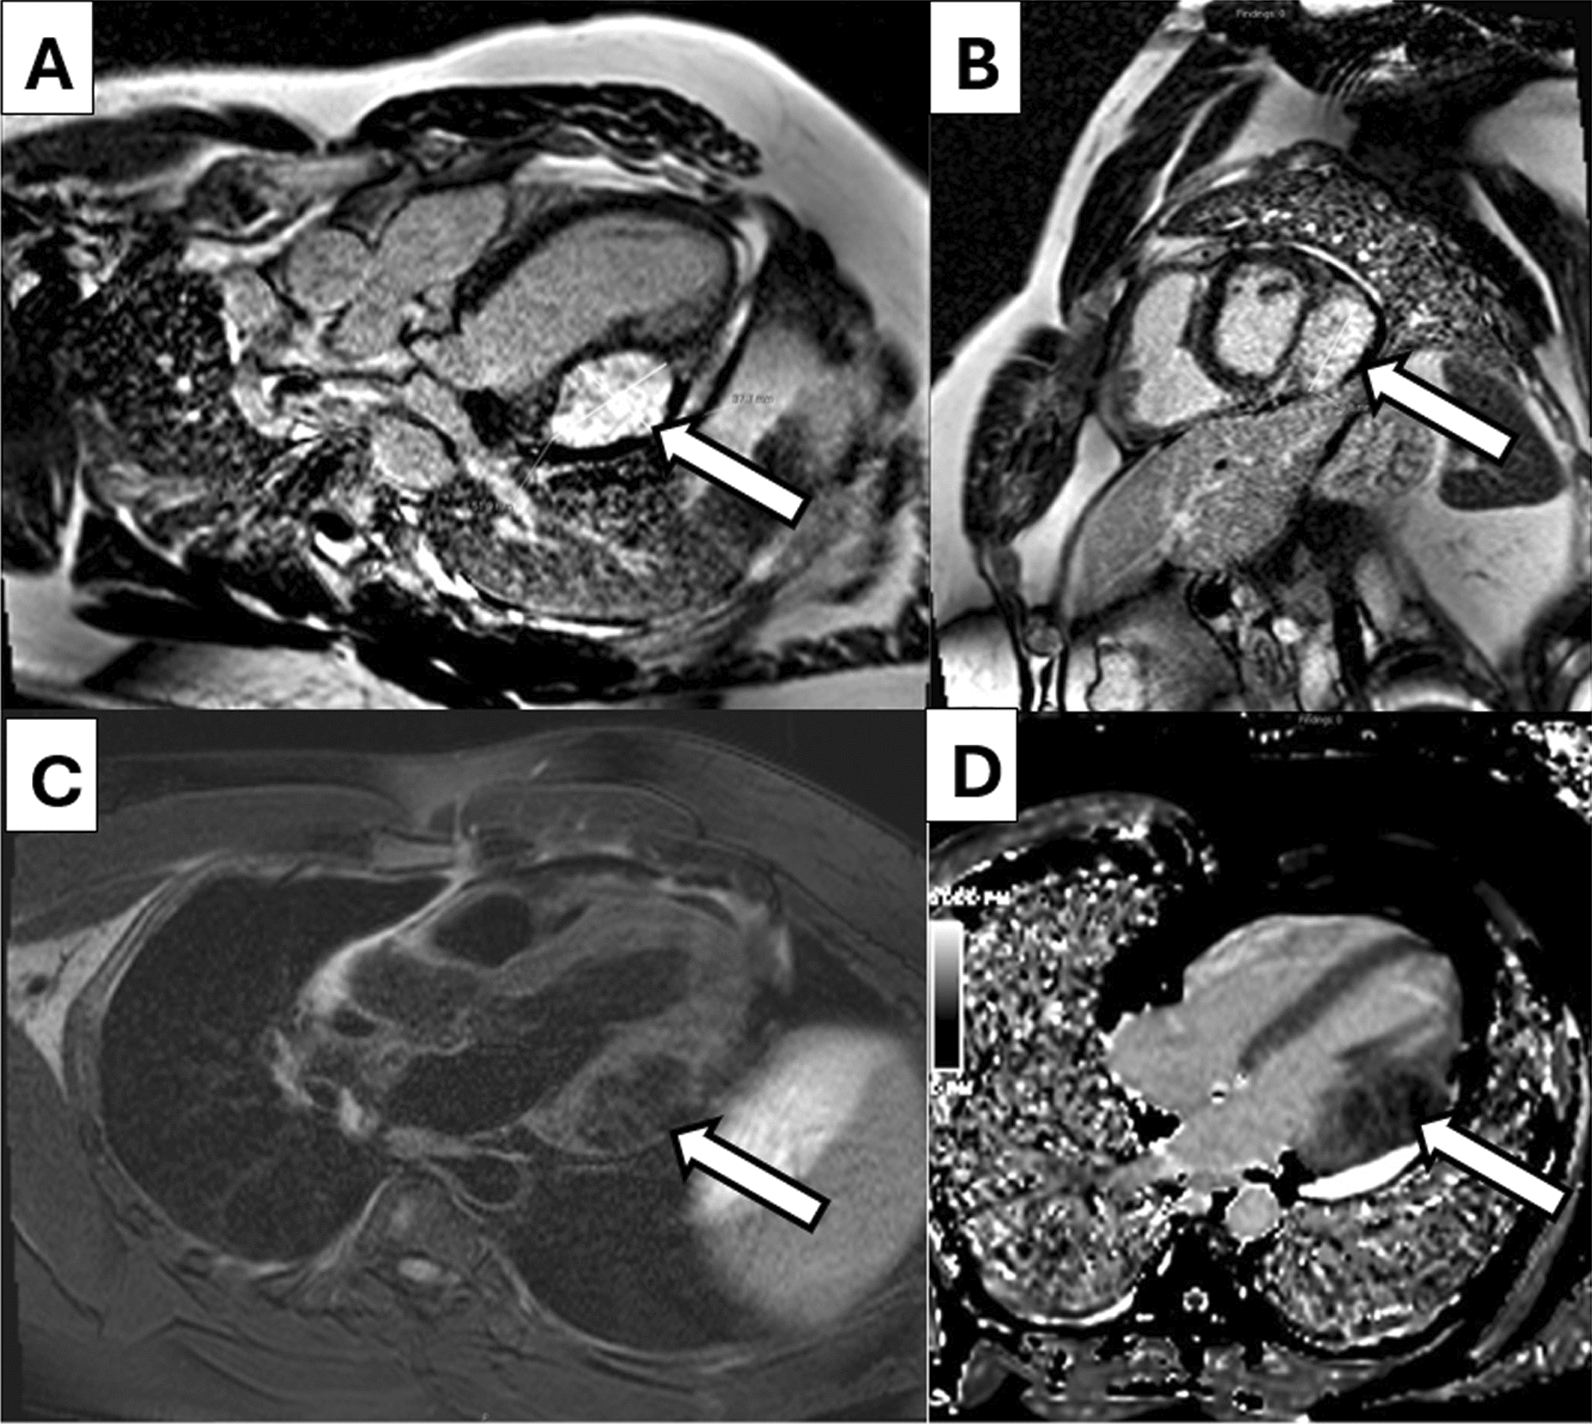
Repeat MRI Images: A repeat MRI was obtained for sizing and evaluation of the mass with the mitral valve apparatus. On late gadolinium enhancement (LGE) images, the mass (arrows) has extensive LGE avidity A, B and measured 5.2 × 5.1 × 3.8 cm. On T1 C and T2 D weighted imaging, there did not appear to be involvement of the mitral valve appartatus and the mass appeared well circumscribed compared to native myocardium
